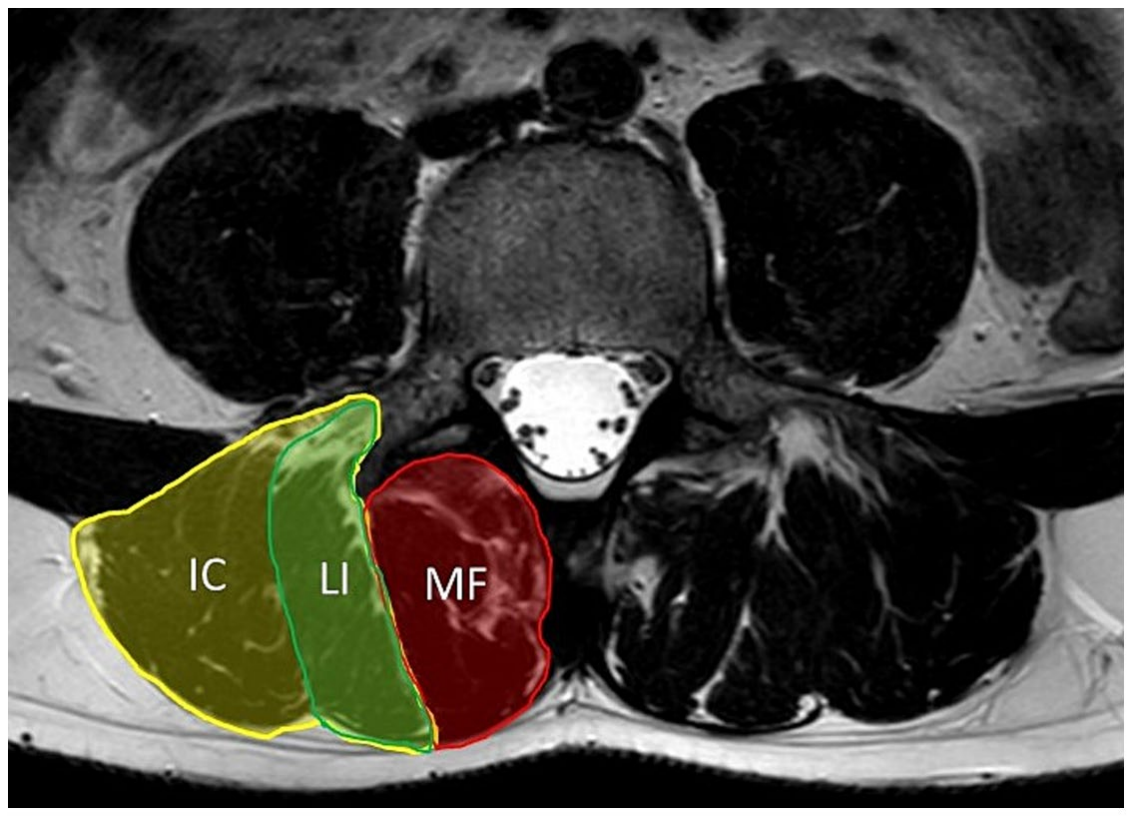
Axial T2-weighted MRI of lumbar spine at level of L4. Figure shows anatomy of lumbar paraspinal muscles. The erector spinae muscle consists of the longissimus (LI) and iliocostalis (IC) muscles. The multifidus muscle (MF) is medial to the erector spinae muscle.
